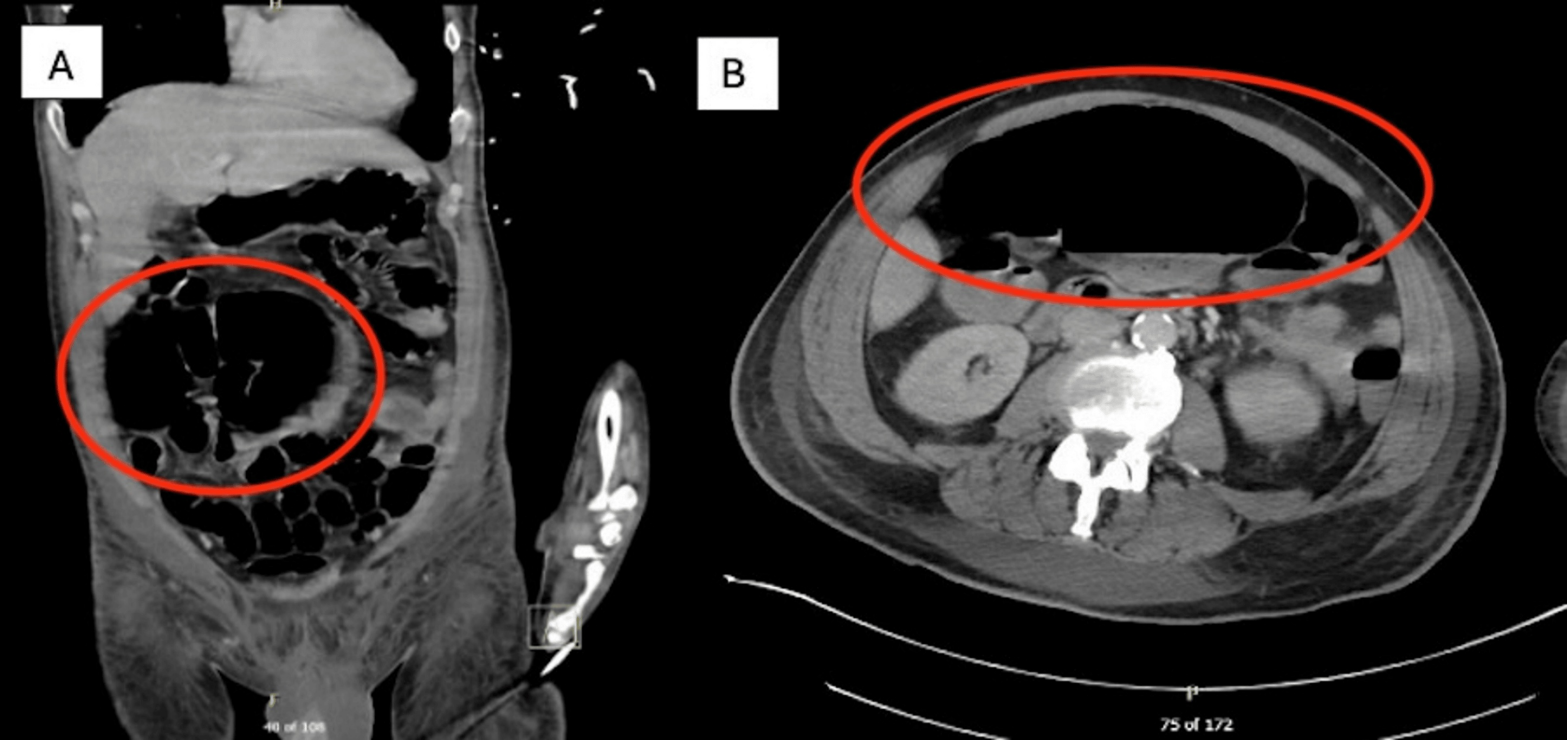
Computed tomography scan of the abdomen and pelvis Computed tomography scan of the abdomen and pelvis, completed on 2/11/2024, includes coronal (A) and axial (B) views. Gaseous distension of the cecum is seen in (A) and (B), as depicted by a red circle.
